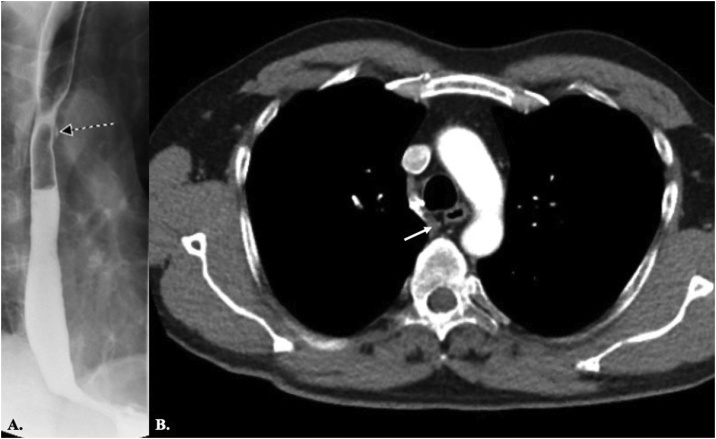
A) The barium swallow study shows an external compression over the esophagus (black arrow). B) Chest CT before treatment shows vascular ring and aberrant right subclavian artery with mass effect over and deviation of the esophagus (white arrow).
